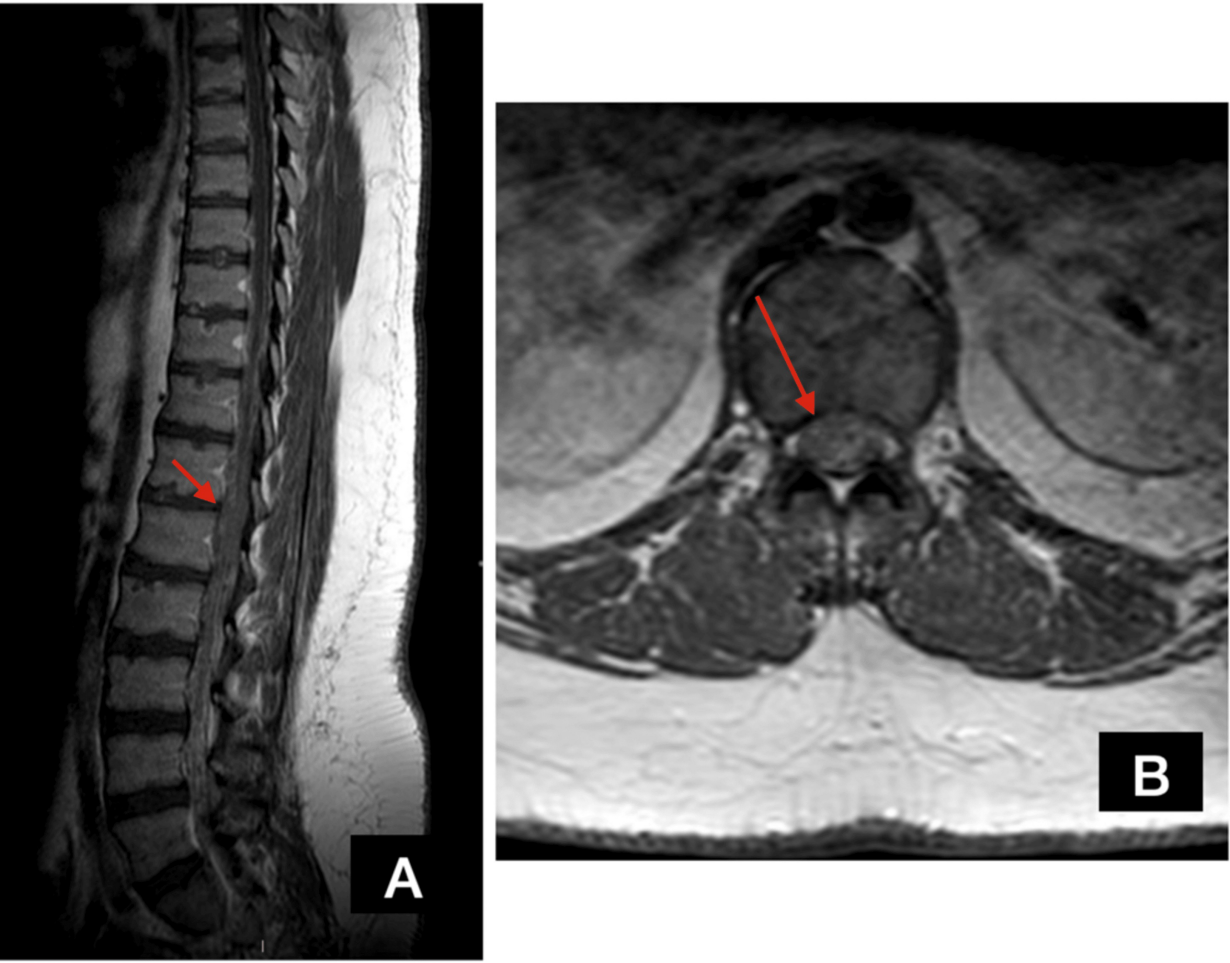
Post-op MRI scan findings. A: sagittal post-op, post-contrast T1 MRI scan; B: axial post-op post-contrast T1 MRI. Both scans demonstrate the complete removal of the lesion.
